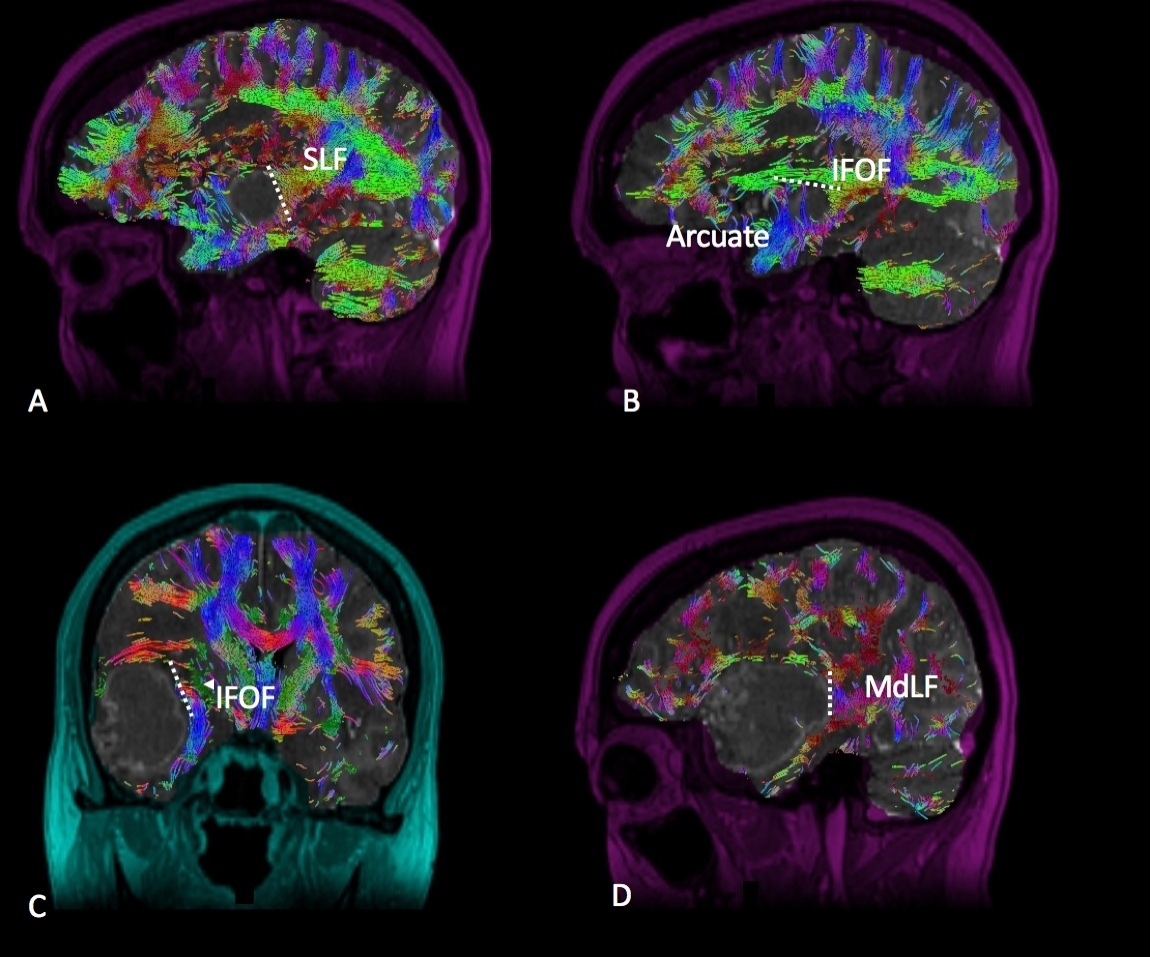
Posterior Temporal Diffusion tractography enhanced T1 magnetic resonance imaging (MRI) with contrast with (A) sagittal, (B) sagittal, (C) coronal, and (D) sagittal views demonstrating a posterior temporal glioma in relation to the surrounding white matter tracts. Labeled white matter tracts associated are shown as follows: inferior fronto-occipital tract (IFOF), superior longitudinal fasciculus (SLF), middle longitudinal fasciculus (MdLF), and arcuate fasciculus (Arcuate). The disconnection pattern is shown as a white dotted line.
